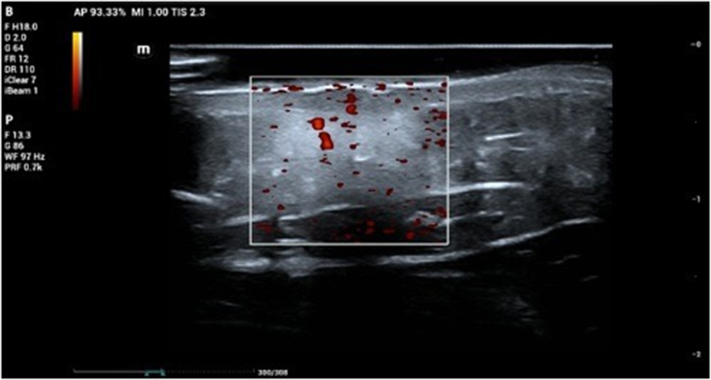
High-frequency ultrasound: dermal and subcutaneous lesion with vascular flow on power Doppler.
